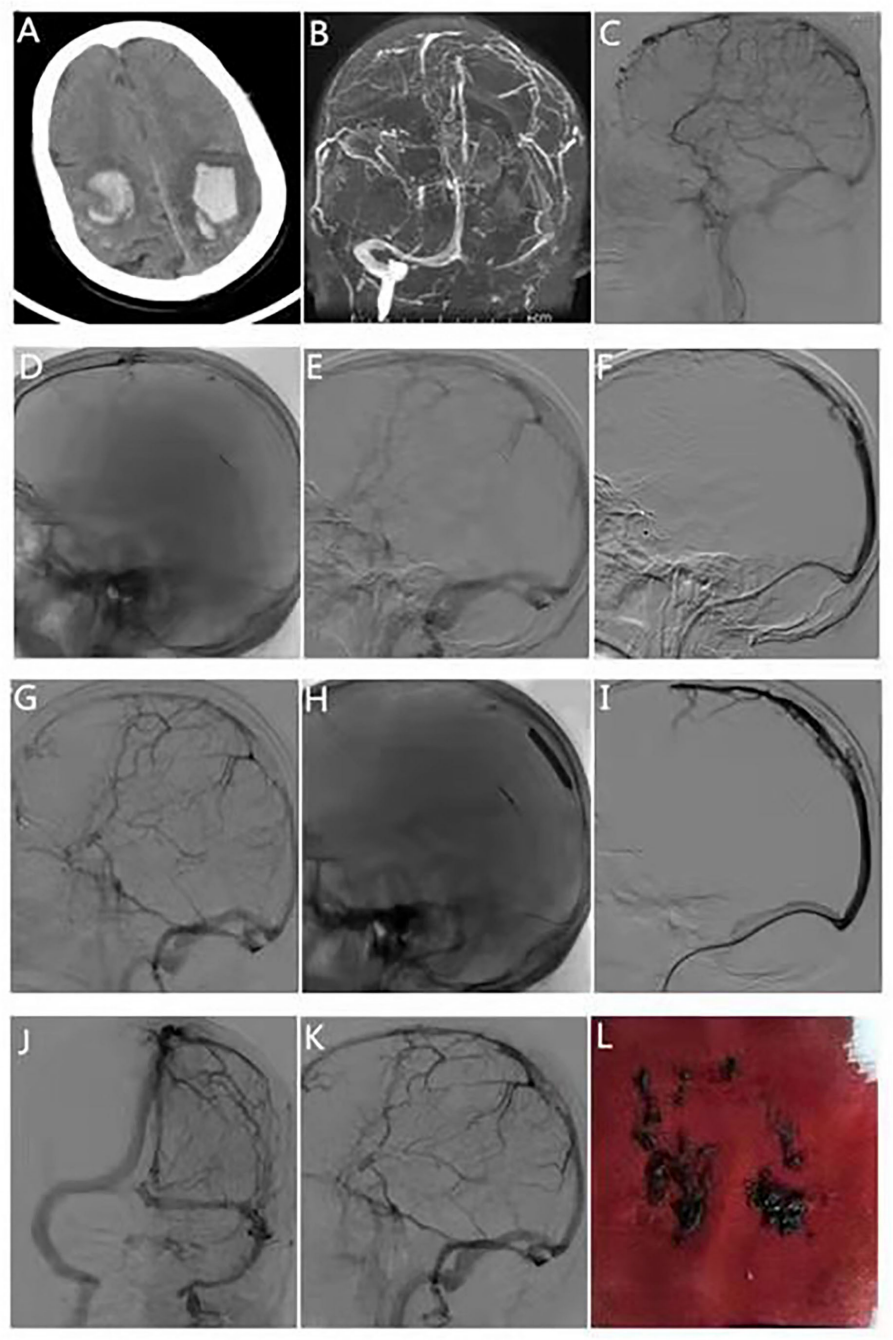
Typical case 1. The patient was a 66-year-old man, transferred from the outside hospital due to sudden unconsciousness, limb weakness, and episodic limb convulsions for 5 days. (A) Head computed tomography scan at the time of transfer shows bilateral parietal hemorrhage. (B) Magnetic resonance venography at the time of admission shows occlusion of the superior sagittal sinus. (C) Digital subtraction angiography shows occlusion of the middle and proximal sagittal sinus. (D) Microcatheter angiography suggests patency of the distal segment of the superior sagittal sinus. (E) Stent retrieval was performed for interruption of the superior sagittal sinus. (F) Mechanical thrombectomy of the middle segment of the superior sagittal sinus and delivery of the intermediate catheter into the proximal end of the superior sagittal sinus using the stent anchoring technique. (G) Residual stenosis in the middle segment of the superior sagittal sinus after thrombectomy of the middle segment and aspiration of the intermediate catheter. (H) Balloon dilatation of the middle segment of the superior sagittal sinus. (I) Intermediate catheter retrograde angiography suggests that the superior sagittal sinus is normal, stenosis is relieved. (J,K) Intermediate catheter retrograde angiography suggests that the superior sagittal, bilateral transverse, and sigmoid sinuses are well-visualized. (L) Venous thrombus has been removed.
